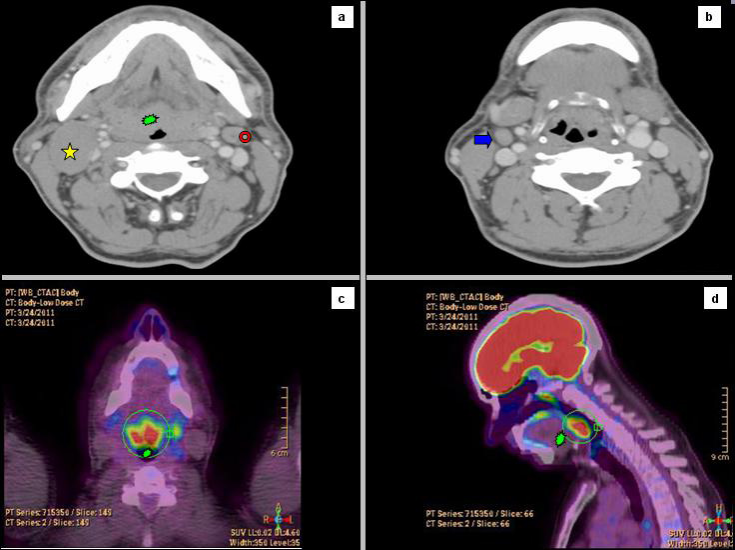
Enlarged level Ib (star) and IIA (arrow) right nodes (Figure 1a-b) and level IIA left node (circle; Figure 1a) with thickening of the base of the tongue (blast; Figure 1a) at diagnostic CT scan; base of the tongue hyperaccumulation at 18-FDG- PET scan (Figure 1c-d).
